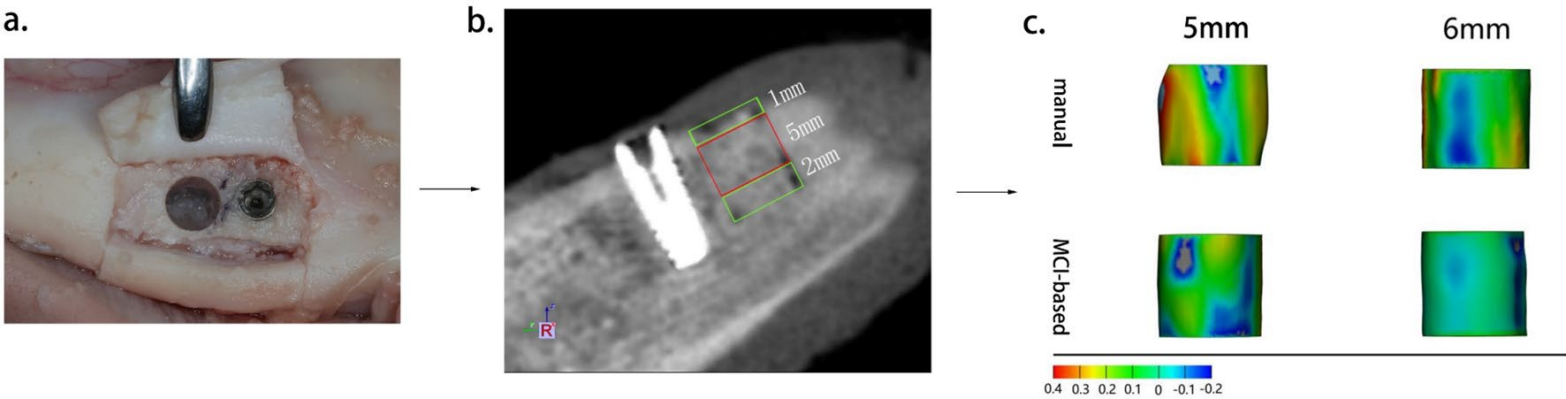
(a) Occlusal view of a cylindrical peri-implant bone defect. (b) CBCT images with the volumetric measurements of the 5 mm height of bone grafts in the augmented regions. (c) 3D color map showing morphological deviation between the 5 mm height of the ideal cylinder and the 3D surface renderings using manual and MCI-based segmentation.
